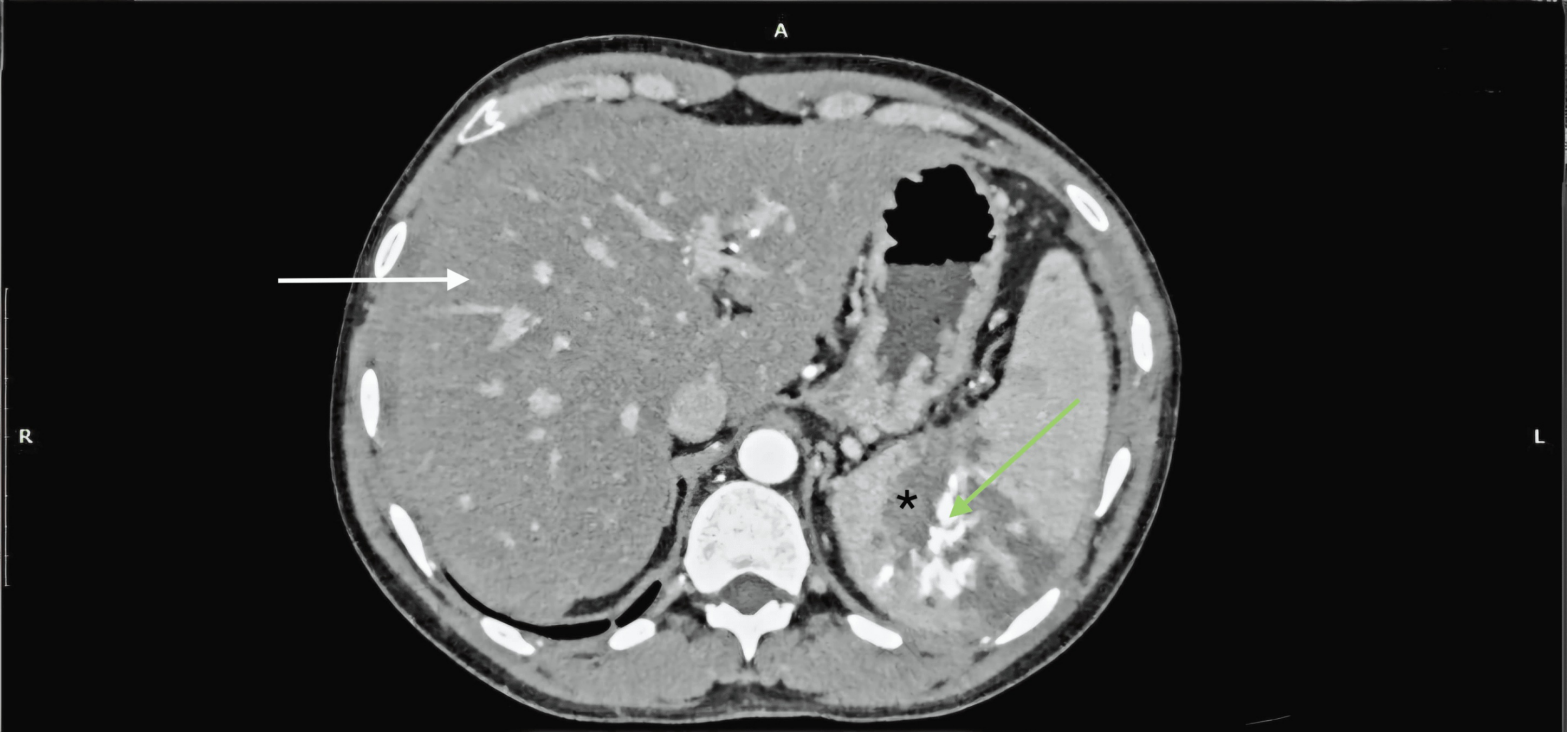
AAST grade IV splenic injury with parenchymal laceration and active contrast extravasation on CECT (SB protocol). A 33-year-old male with a history of RTA underwent CECT of the abdomen using the SB protocol. The axial CECT image shows a massive parenchymal laceration extending to the splenic hilum (black star), with active contrast extravasation (green arrow) confined within the splenic capsule, consistent with the AAST grade IV splenic injury. An incidental note was made of diffusely reduced attenuation of the liver parenchyma (white arrow), suggestive of fatty infiltration, which led to reduced enhancement. AAST: American Association for the Surgery of Trauma; CECT: contrast-enhanced computed tomography; SB: split-bolus; RTA: road traffic accident.
